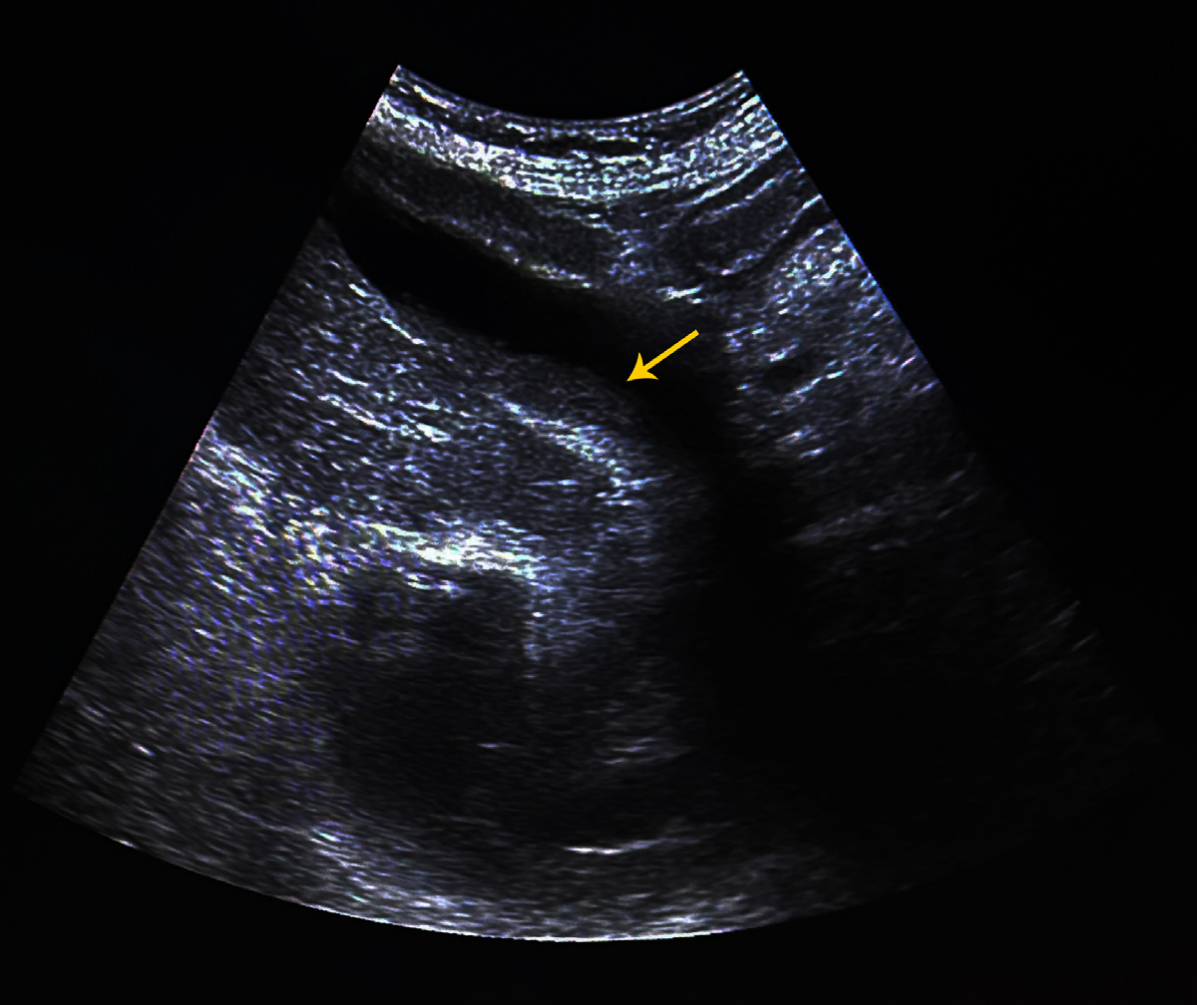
Abdominal ultrasound showing a longitudinal view of the dysmorphic gallbladder. A fine, hyperechogenic, non-shadowing material is seen layering dependently within the lumen (yellow arrow), consistent with biliary sludge.
